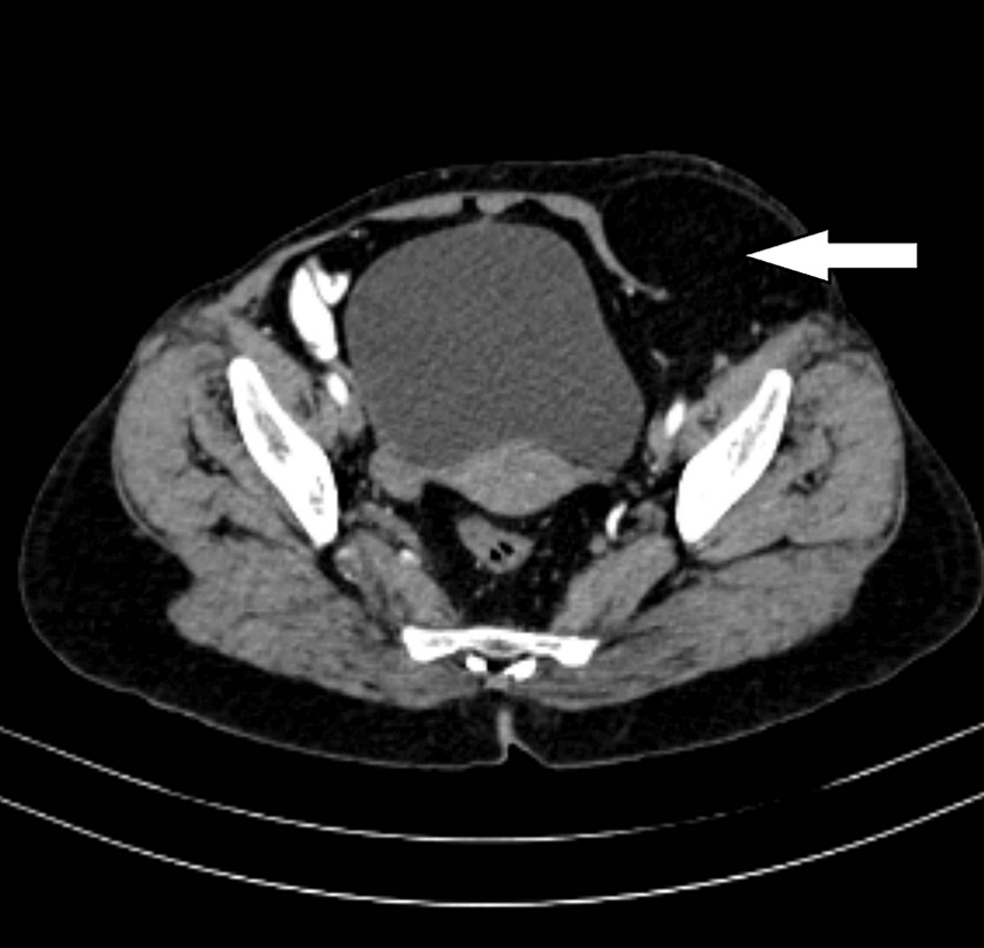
Computed tomography showing the outpouching of bowel loops The white arrow indicates outpouching of the bowel loops through the anterior abdominal wall
